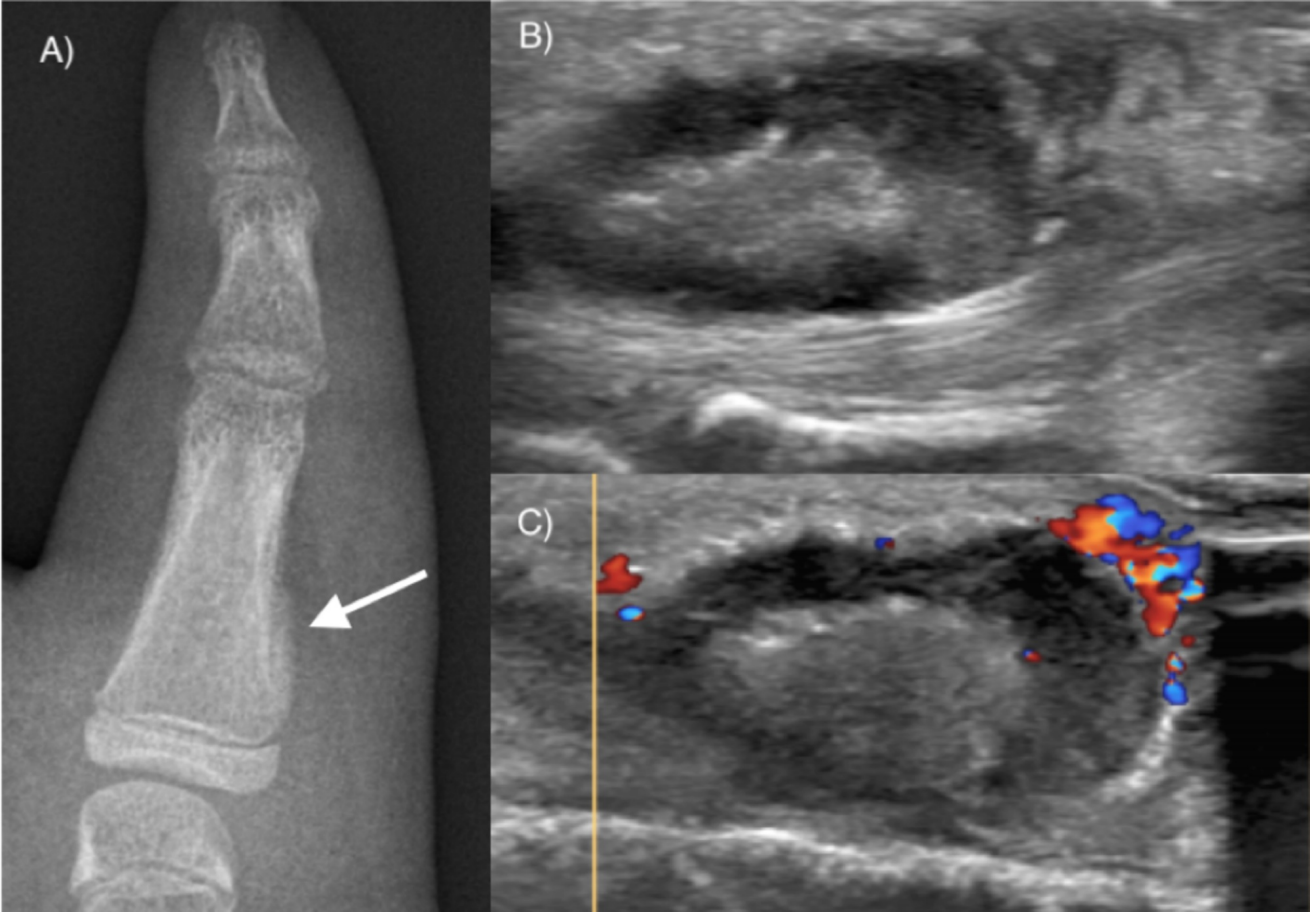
Representative radiologic and ultrasonographic findings A) radiograph demonstrating periosteal reaction (white arrow) in the ulnar aspect of the proximal phalanx of the fifth finger; B) ultrasound B-mode imaging shows a central echogenic region, with a hypoechoic rim; C) Doppler interrogation demonstrates minimal vascularity associated with the hypoechoic region, as well as surrounding hyperemia
